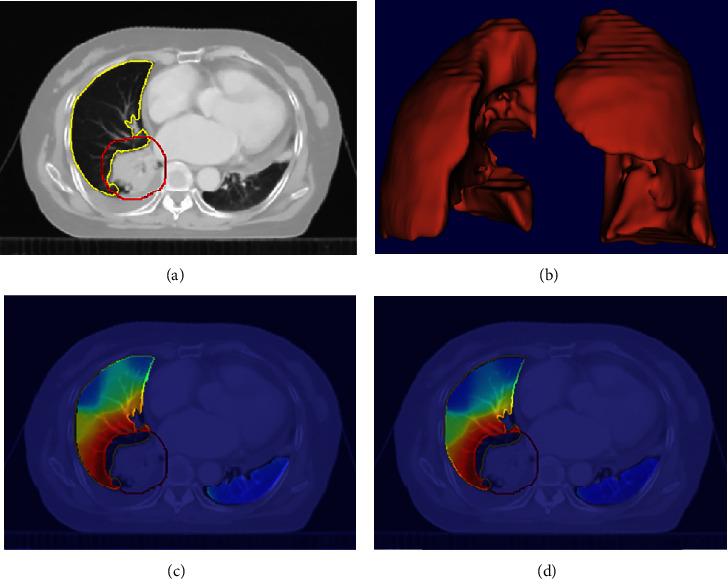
The results of data acquisition and dose conversion: (a) the delineated CTV and lung in CT image, the red is CTV, and the yellow is lung; (b) the 3D volume of lung-CTV; (c) the overlapping of physical dose distribution and CT image; (d) the overlapping of EQD_2_ distribution and CT image.
